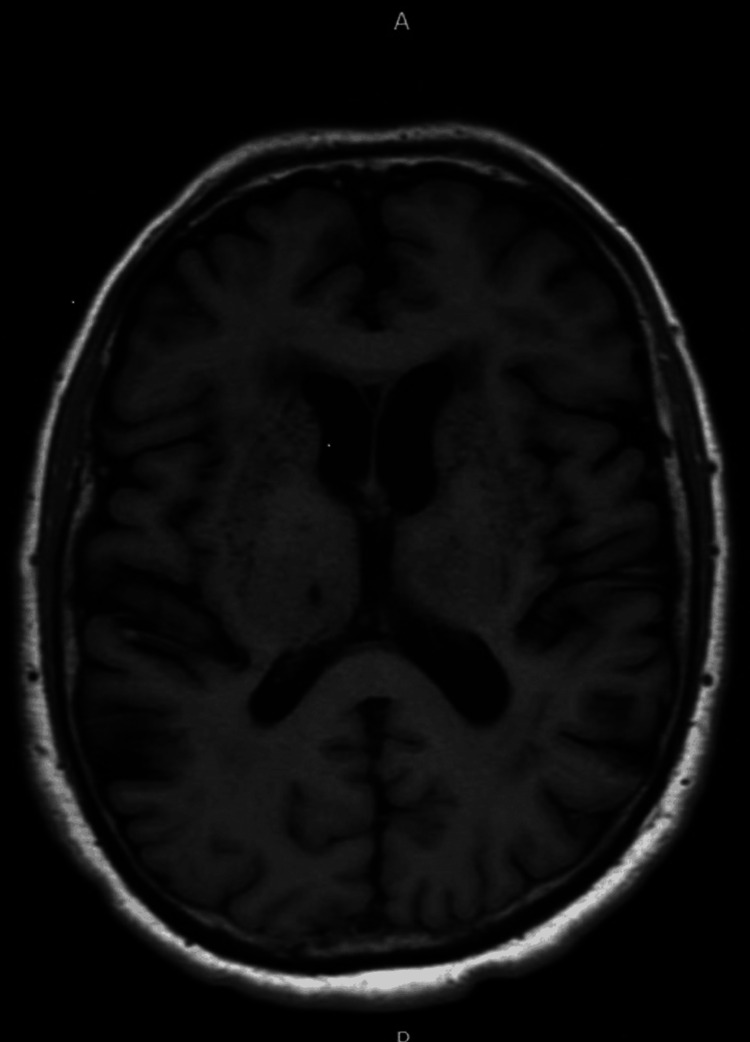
MRI of the brain without contrast with T1 attenuated sequence shows severe microvascular ischemic disease with several chronic lacunar infarcts bilaterally in the basal ganglia and in the right thalamus. MRI: magnetic resonance imaging
